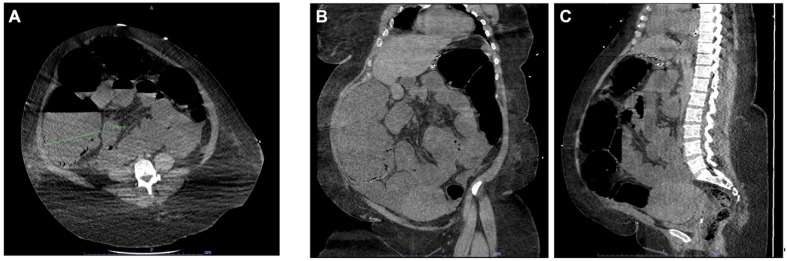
(A) Abdominal CT scan axial section showing the massive dilation of the caecum; (B) CT scan coronal section showing the dilation of the entire colon; (C) sagittal view.
